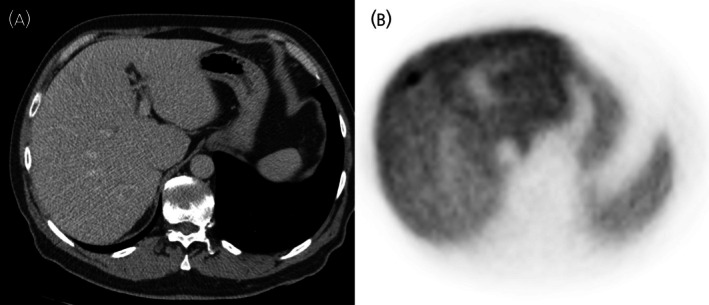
(A) In Patient 42, the liver metastasis on CT was undetectable. (B) Whereas, HED‐PET/CT showed distinct uptake in the metastasis.
